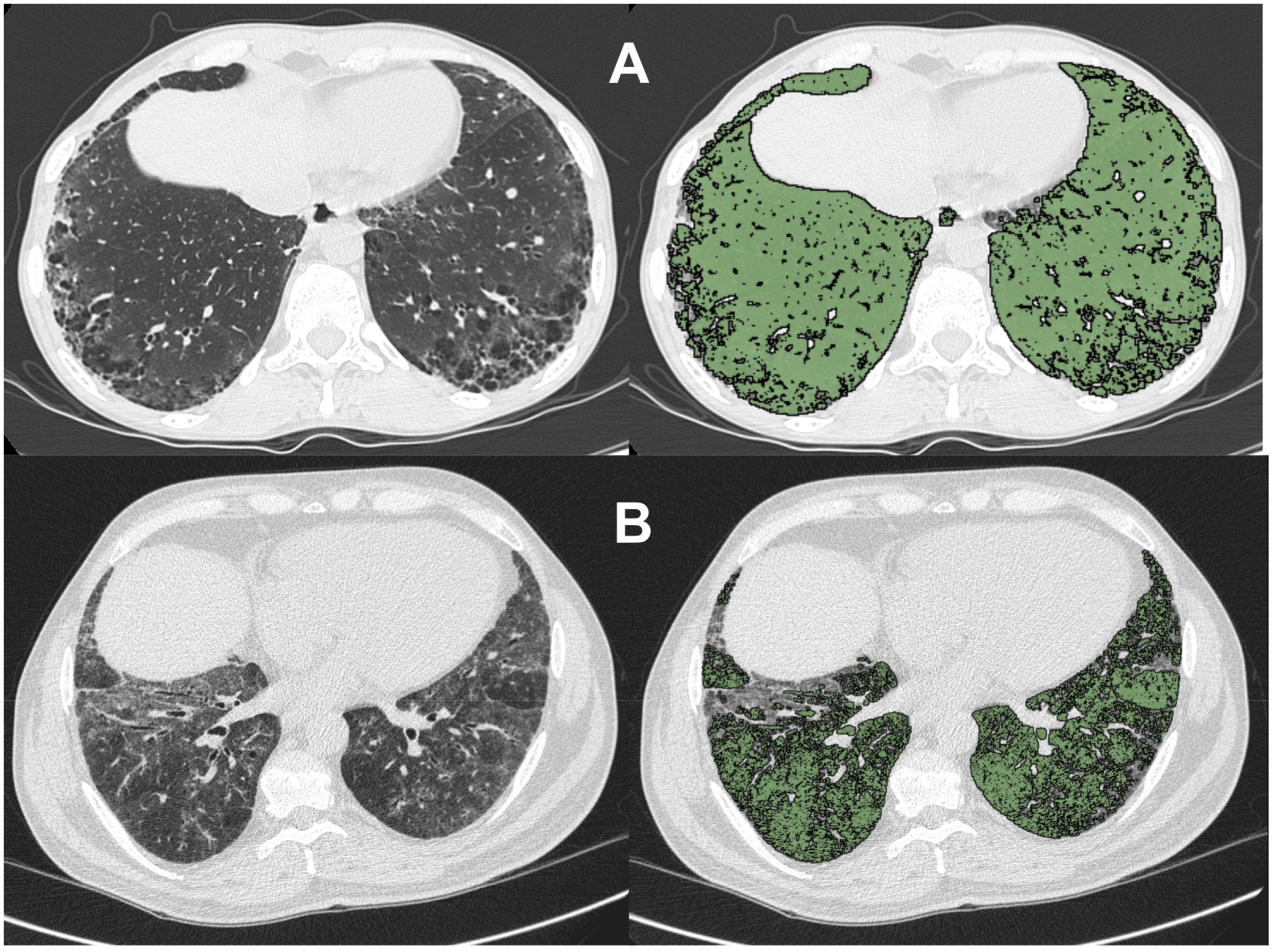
Representative sequences of the OsiriX segmentation process of two study participants. (A) Patient with mild (13%) of pulmonary fibrosis. (B) Patient with severe (41%) pulmonary fibrosis. We have developed the pulmonary fibrosis fraction by the following formula: total HRCT lung volume (−1.024 to −200)–nonfibrotic HRCT lung volume (−1.024 to –700) divided by total HRCT lung volume (−1.024 to −200) multiplied by 100.
